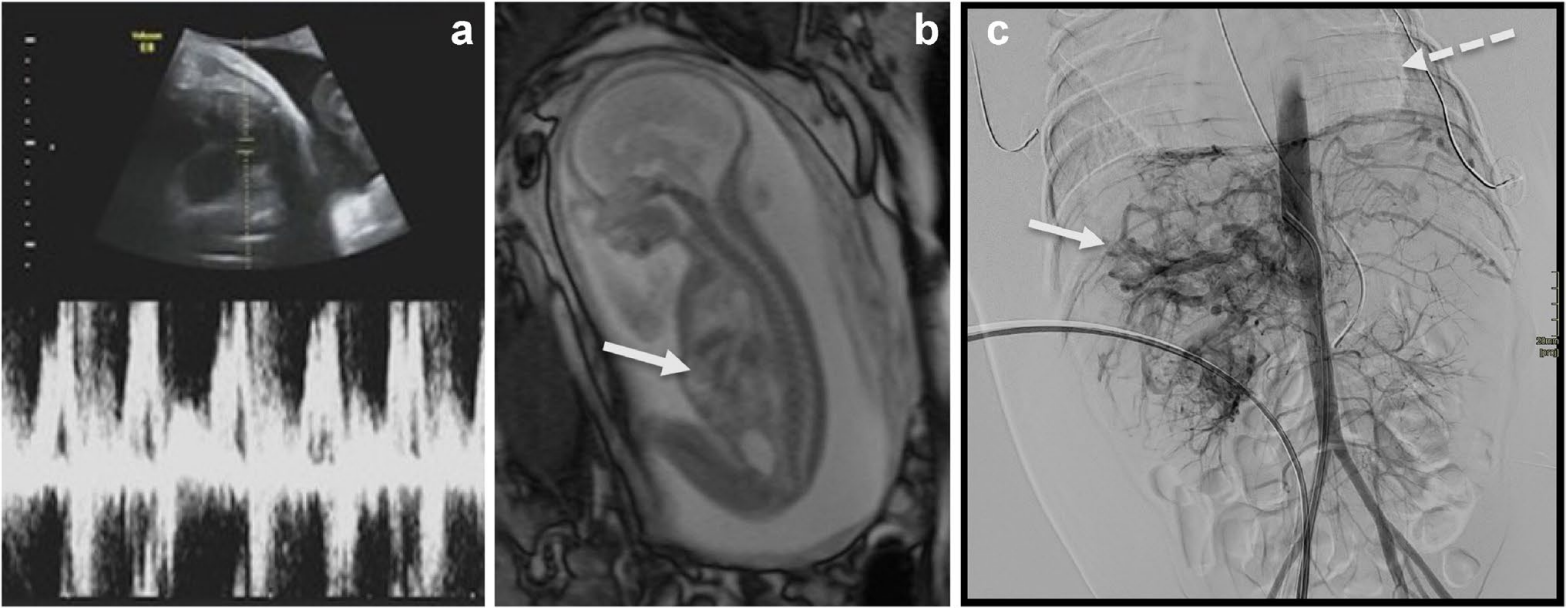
Arteriovenous malformation of the liver in a female fetus at 23 weeks gestational age. a Prenatal oblique ultrasound demonstrates high-flow signal in the liver. b Fetal sagittal T2-weighted magnetic resonance image demonstrates the high-flow signal voids in the liver (*arrow*), without soft-tissue mass, differentiating this lesion from congenital hemangioma. c Postnatal coronal angiographic image of this high-flow lesion (*arrow*) shows extensive shunting and cardiomegaly (*broken*
